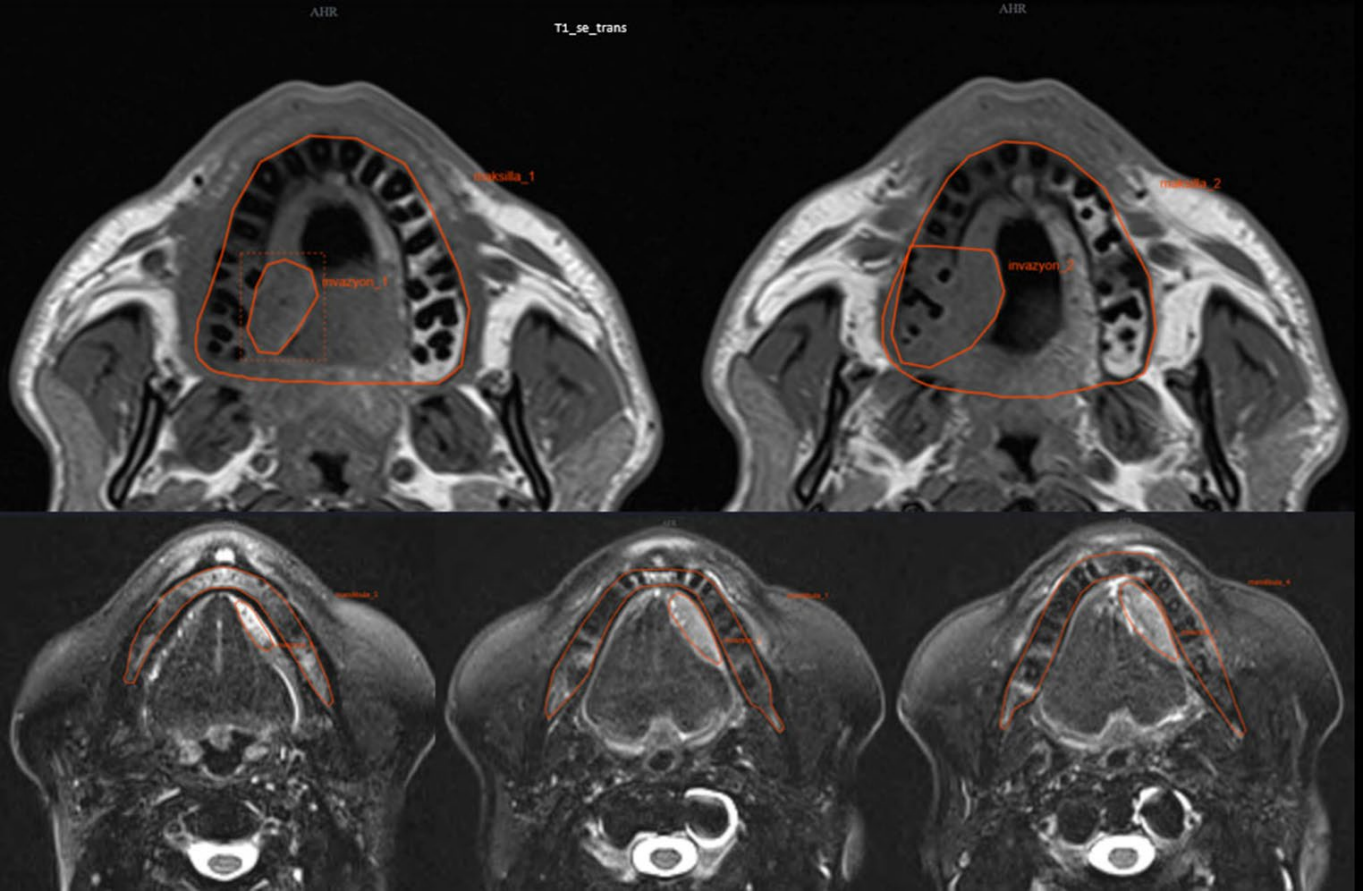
T1-Se axial MR images showing bone invasion area of the maxillary and mandibular bone and oral SCC on T2-TSE-transversal-fat-suppressed MRI images which was manually defined on consecutive MR slices
